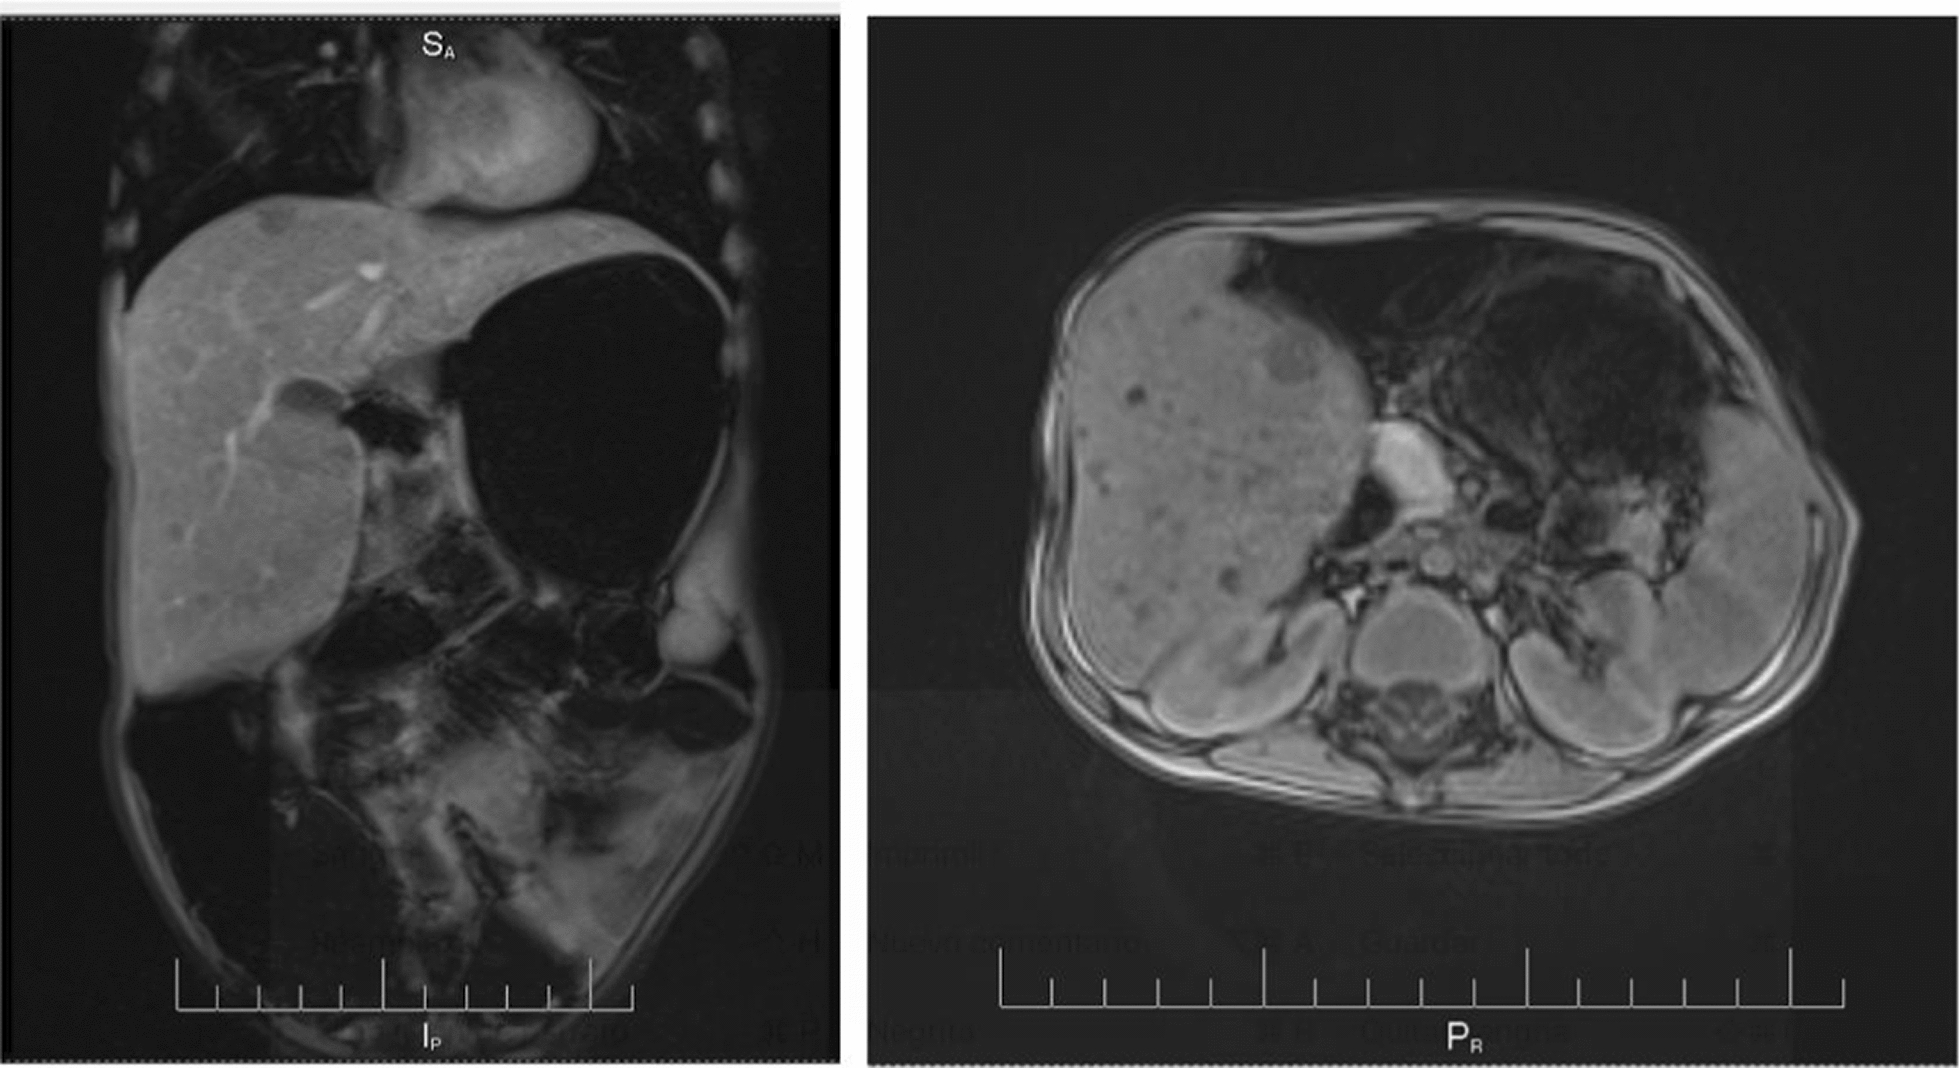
Abdominal MRI after NTBC suspension. Hepatomegaly with solid focal lesions, the largest of which was 11 mm and involved both lobes
